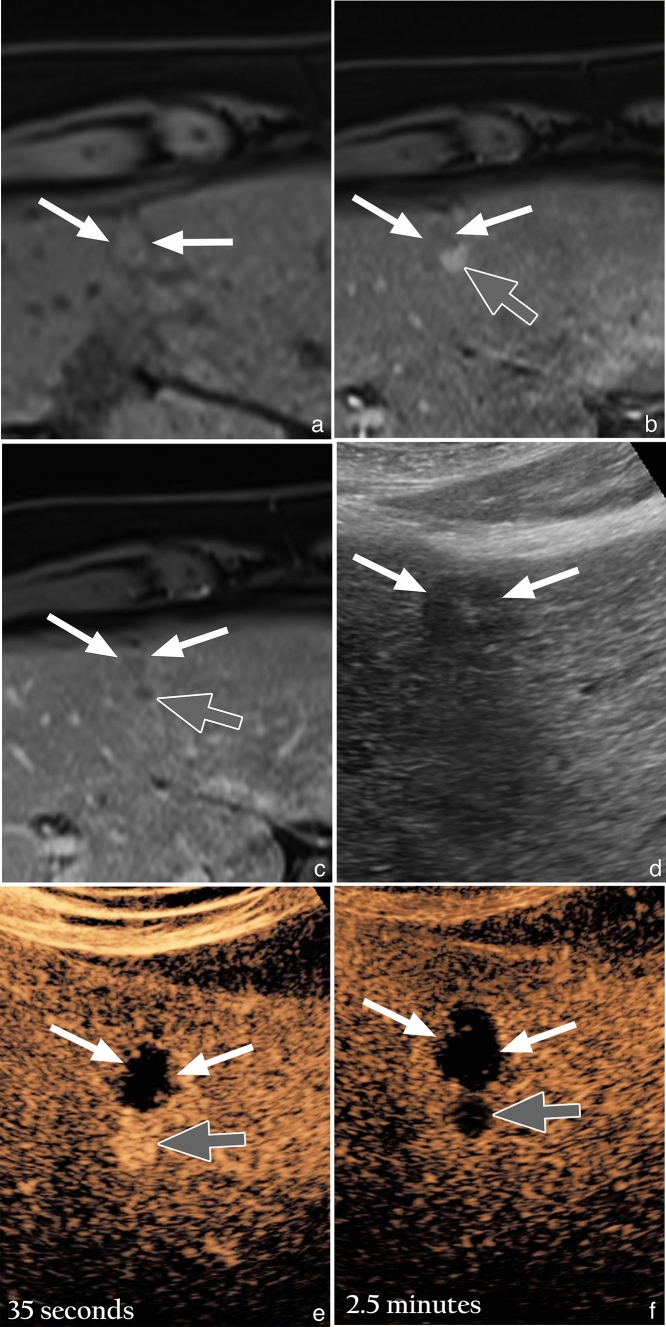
Concordant extrazonal recurrence on MRI and CEUS after MWA in a 54-year-old man with HCV cirrhosis. a) Unenhanced T1 weighted fat suppressed (T1FS) image shows an isointense treatment site (white arrows). b) Arterial phase T1FS subtracted image shows a nodular area of hyperenhancement (gray arrow), inseparable from the posterior margin of the treatment site (white arrows). c) Delayed phase T1FS subtracted image shows washout (gray arrow), corresponding to the nodular area of hyperenhancement seen on the arterial phased image. d) Gray-scale US image, in the same patient, shows a hypoechoic treatment site (white arrows). e) CEUS image obtained 35 seconds after microbubble contrast injection shows a nodular area of hyperenhancement (gray arrow) inseparable from the avascular treatment site (white arrows), equivalent to the arterial phased MR image. f) CEUS image obtained at 2.5 minutes shows definite washout (gray arrow) of the arterially hyperenhancing nodular focus
